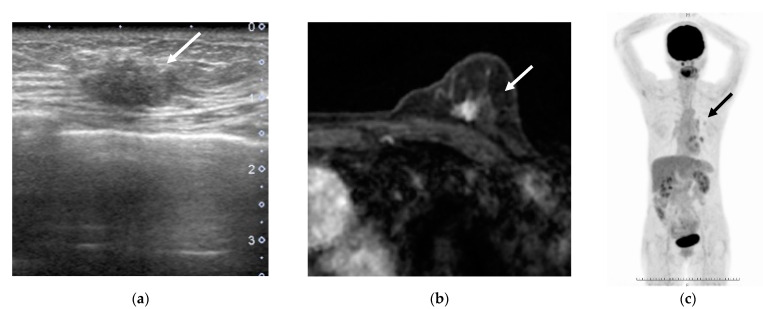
A woman in her 60s was found to have a left invasive ductal carcinoma of no special type by surgery (arrows). (a) Breast ultrasonography (B mode), (b) contrast-enhanced breast magnetic resonance image (axial image of early phase of contrast-enhanced), and (c) whole-body positron emission tomography. Pathological analysis revealed T2N0M0, nuclear grade 1, positive estrogen receptor, positive progesterone receptor, and negative human epidermal growth factor receptor 2 (not shown). She was found to have an SUVmax of 1.4, anatomical stage IIA, clinical prognostic stage IB, and pathological prognostic stage IA.
